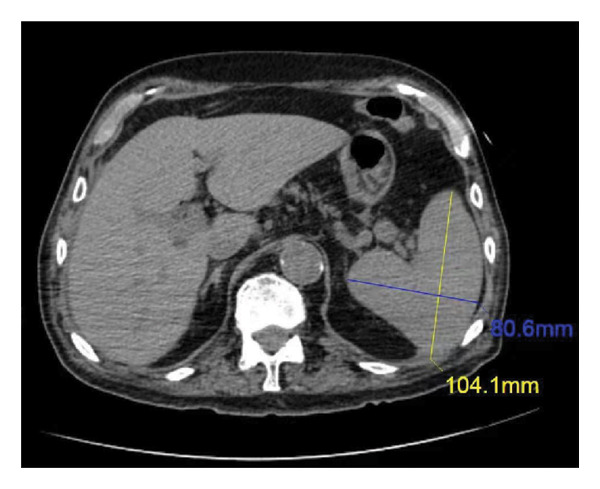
Results of abdominal CT on admission. Abdominal CT showing mild splenomegaly (104.1∗80.6 mm) with an intact capsule and minimal perihepatic and perisplenic fluid.
